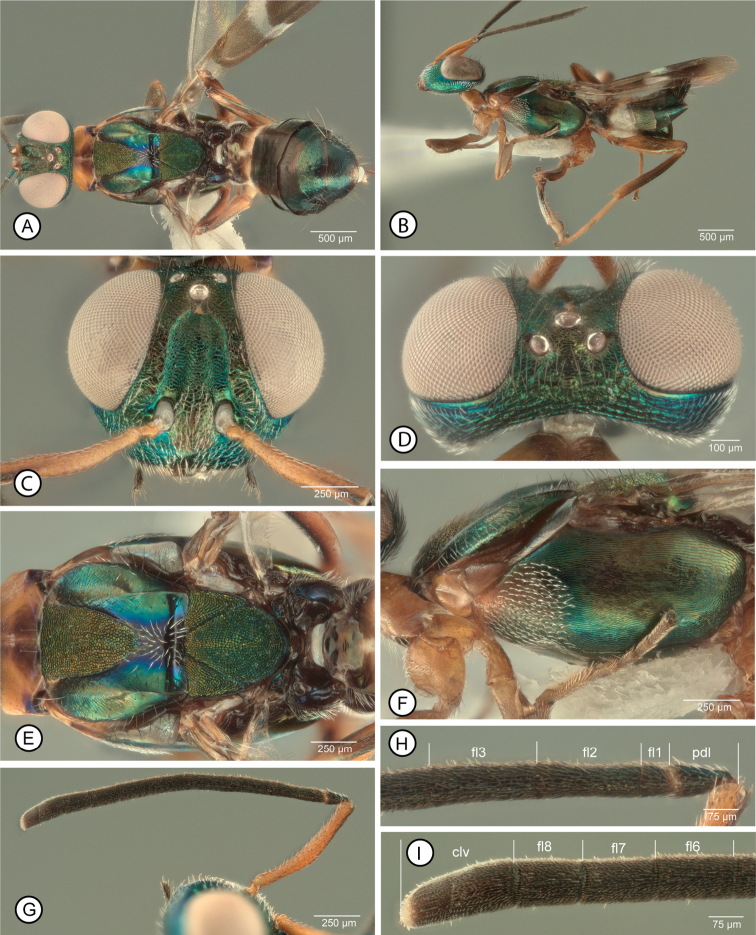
Anastatusgansuensis*, female A dorsal habitus (9) B lateral habitus (7) C head in frontal view (10) D, same in dorsal view (9) E mesosoma in dorsal view (9) F same in lateral view (7) G antenna (10) H pedicel and basal three funiculars (10) I clava and apical three funiculars (10). Abbreviations: clv = clava, flx = flagellomere number; pdl = pedicel
